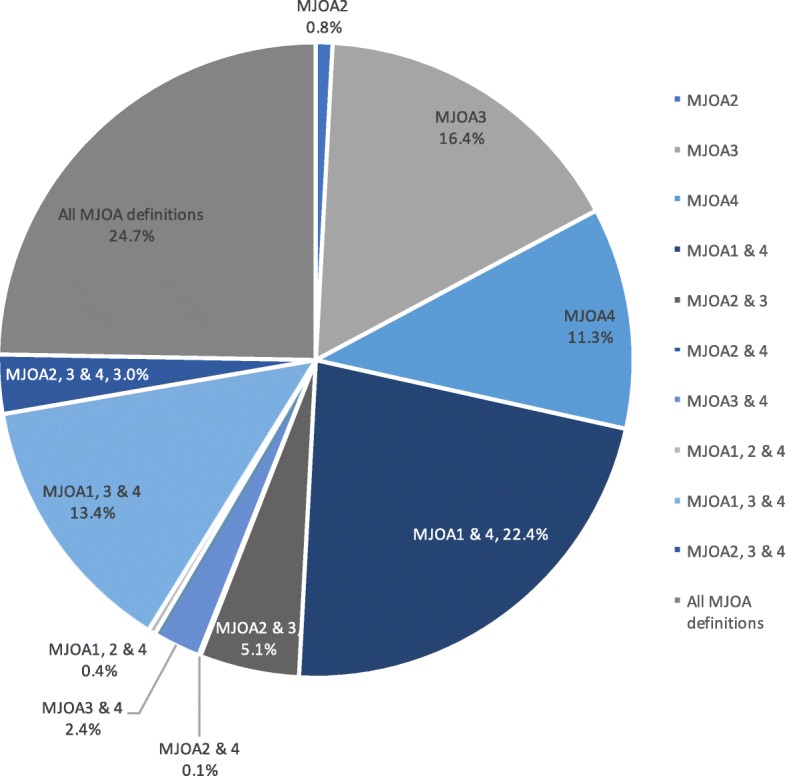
Percentage of those meeting the 4 definitions of radiographic MJOA and their combinations among JoCo OA participants with MJOA (*n* = 1064). Another 613 participants (36.5% of the sample) had no radiographic MJOA, and several combinations were not seen in this cohort (i.e., MJOA-2 only, MJOA-1&2 or 2&3, and MJOA-1,2&3)
